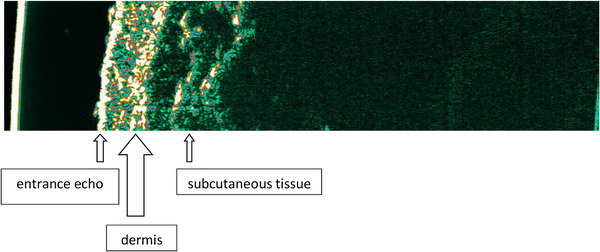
The image of healthy skin in high‐frequency ultrasonography.
